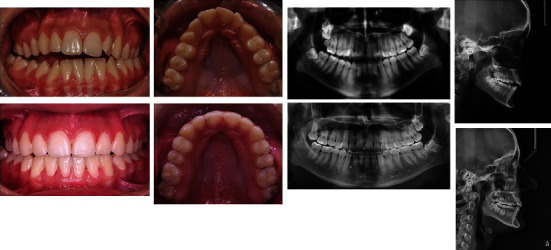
Intraoral photos and X-rays of the initial and finishing condition.
